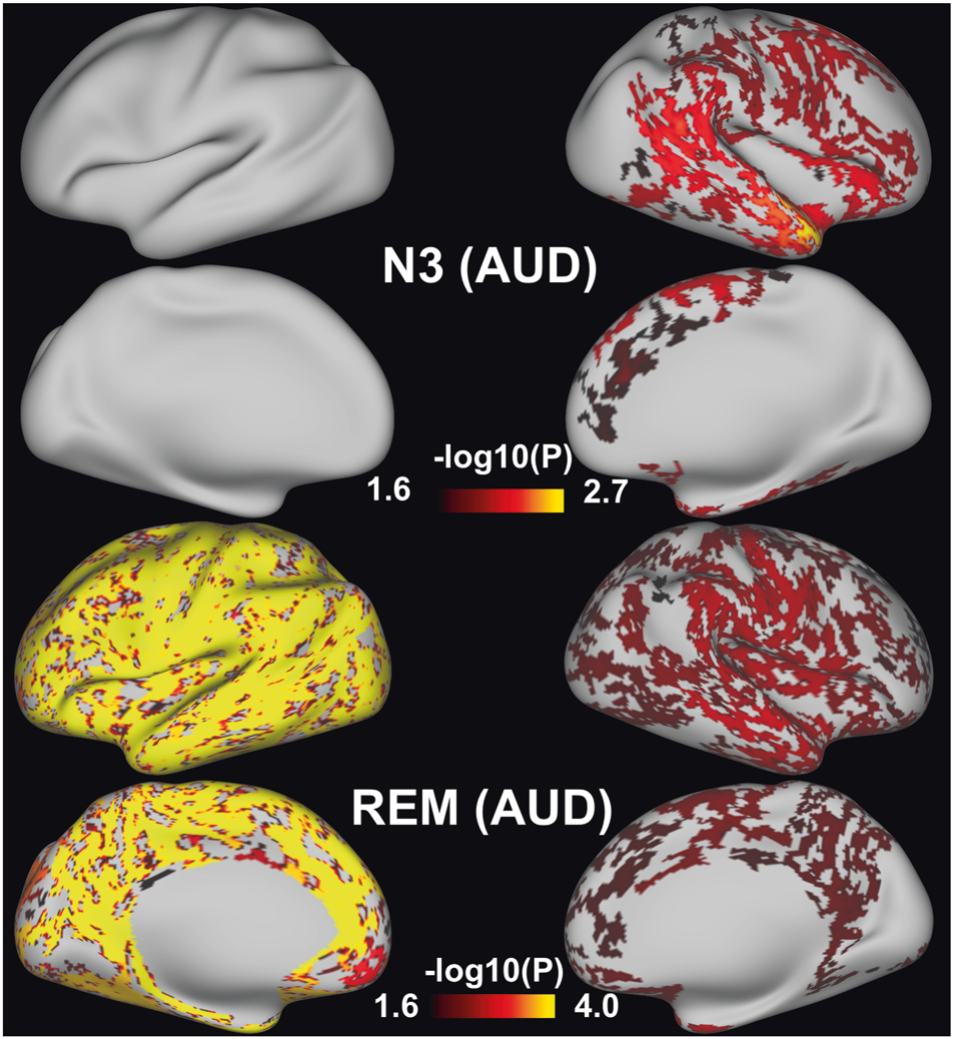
Sleep-CT association in AUD. N3-related and REM-related CT in AUD. The significance threshold was −log10(0.05/2) = 1.602, Bonferroni-corrected for two hemispheres.
